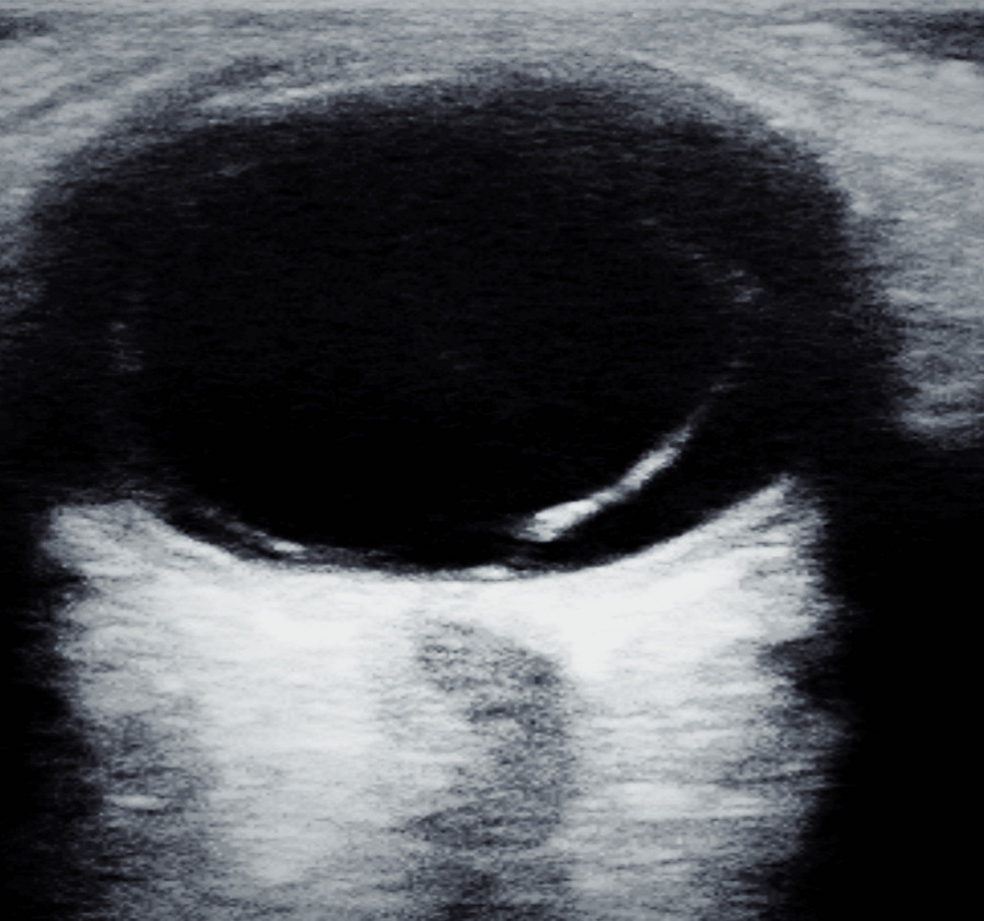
Ultrasound B-scan findings The image showed scleral thickening and the presence of membrane in a dome-shaped manner suggestive of sub-retinal fluid
